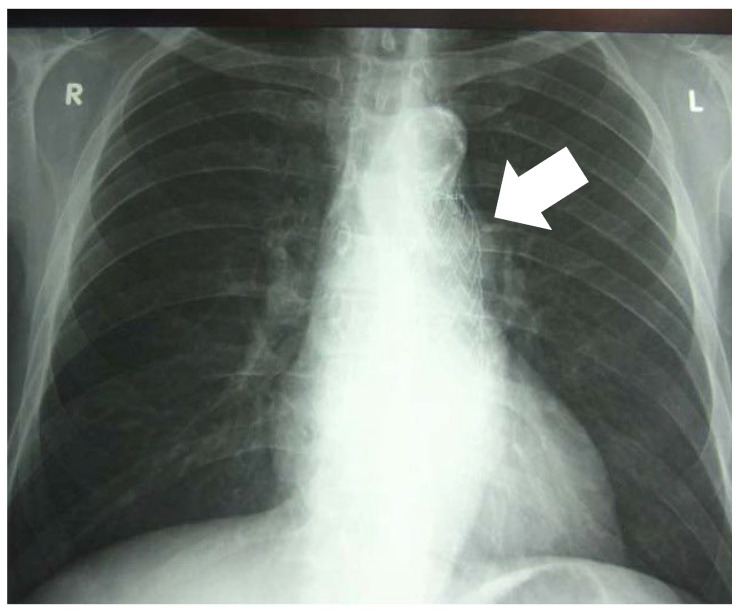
Chest radiography showed no pathological changes in the pulmonary parenchyma. Radiopaque material consistent with prior endovascular intervention was visible in the projection of the thoracic aorta (arrow).
